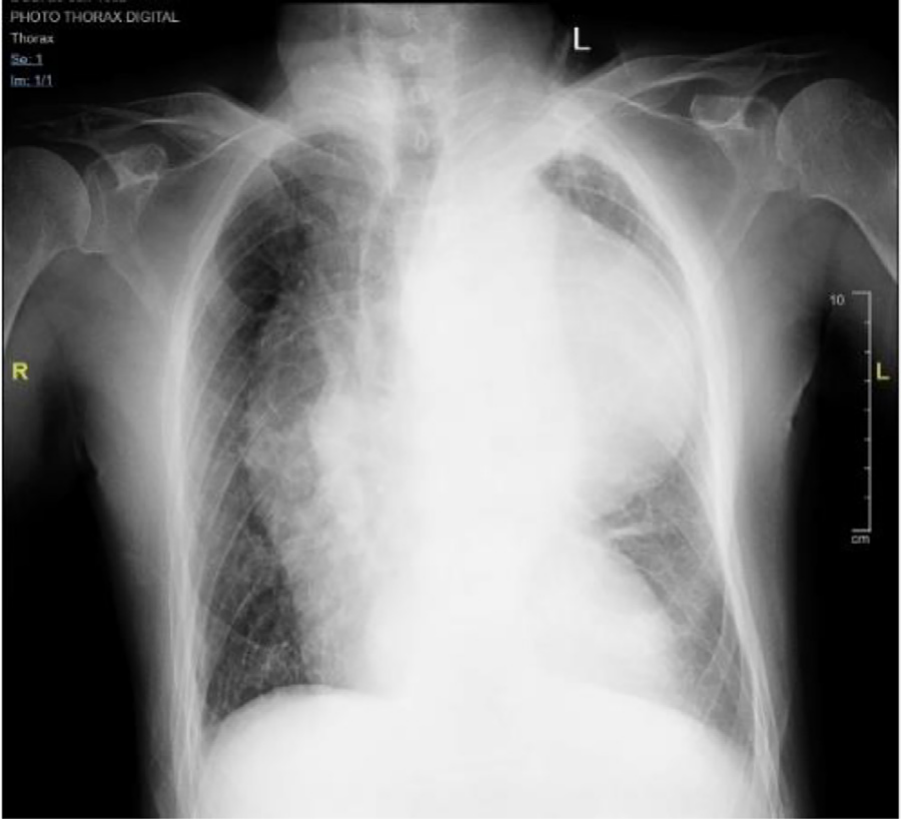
Aneurysm appearance on chest X-ray examination.
